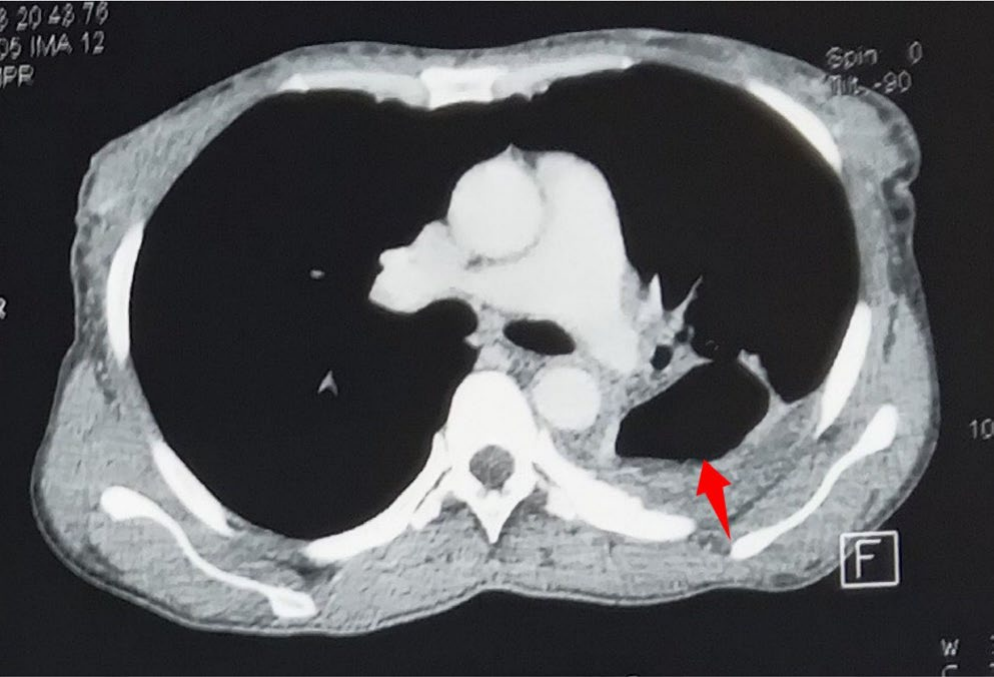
Post‐contrast axial CT scan image of the chest in soft tissue window showing a reduced left upper lobe cavity (red arrow). The Rasmussen aneurysm has completely resolved with normal appearance of the pulmonary vasculature.
